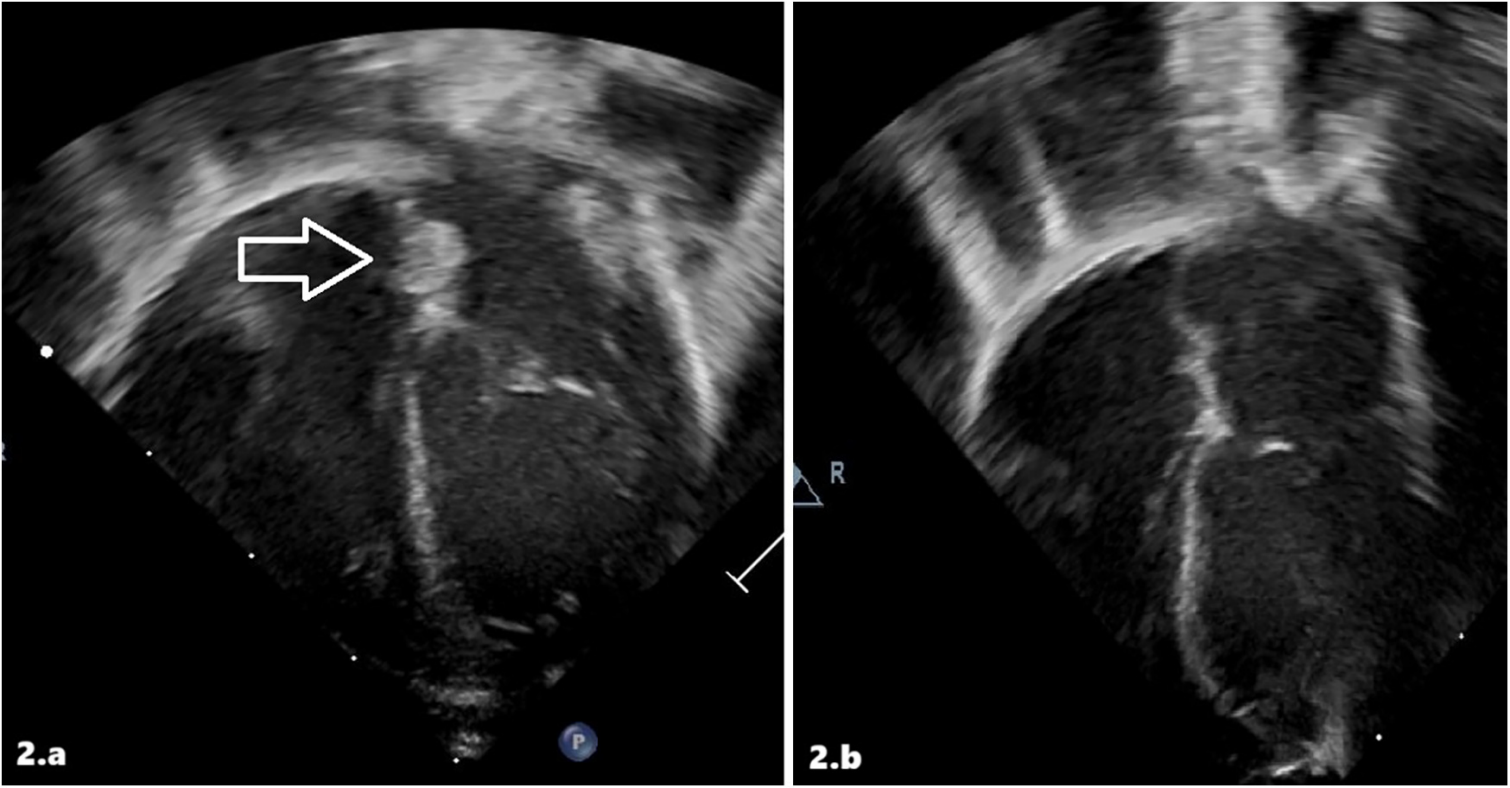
Intracardiac thrombus at diagnosis (a) and after treatment (b) in patient 2 at echocardiographic scans.
